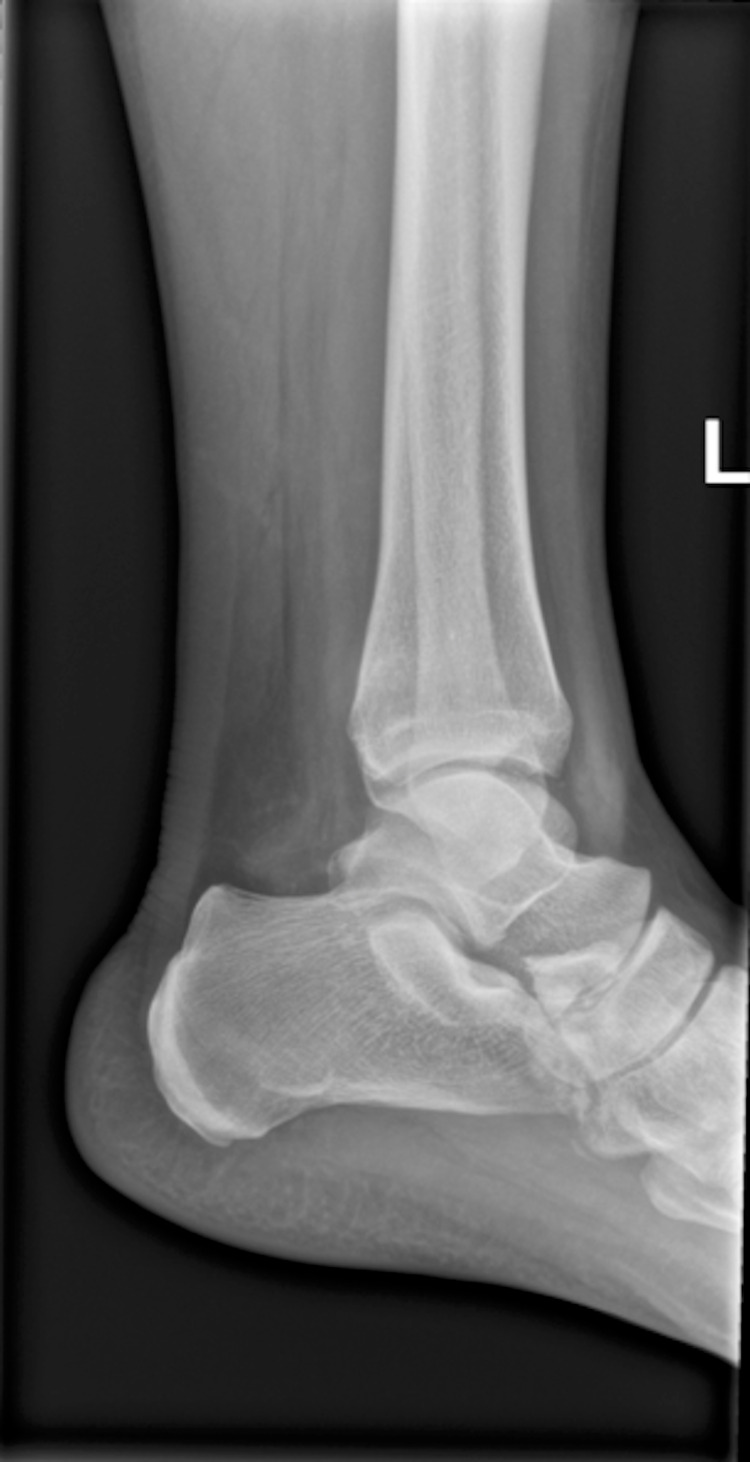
Left ankle lateral radiograph
